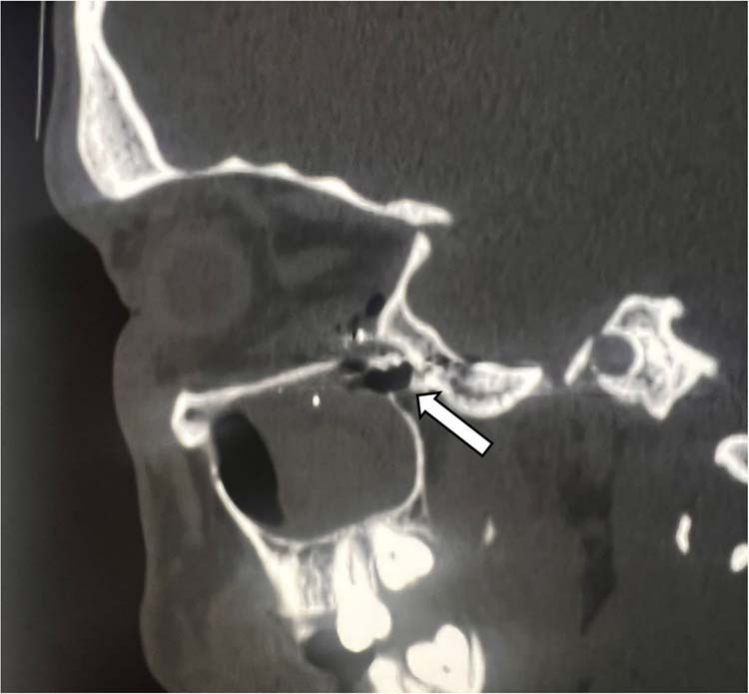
Sagittal section of CT-Head showing multiple shrapnel lodged in the left pterygopalatine fossa resulting fracture of the orbital apex (white arrow).
